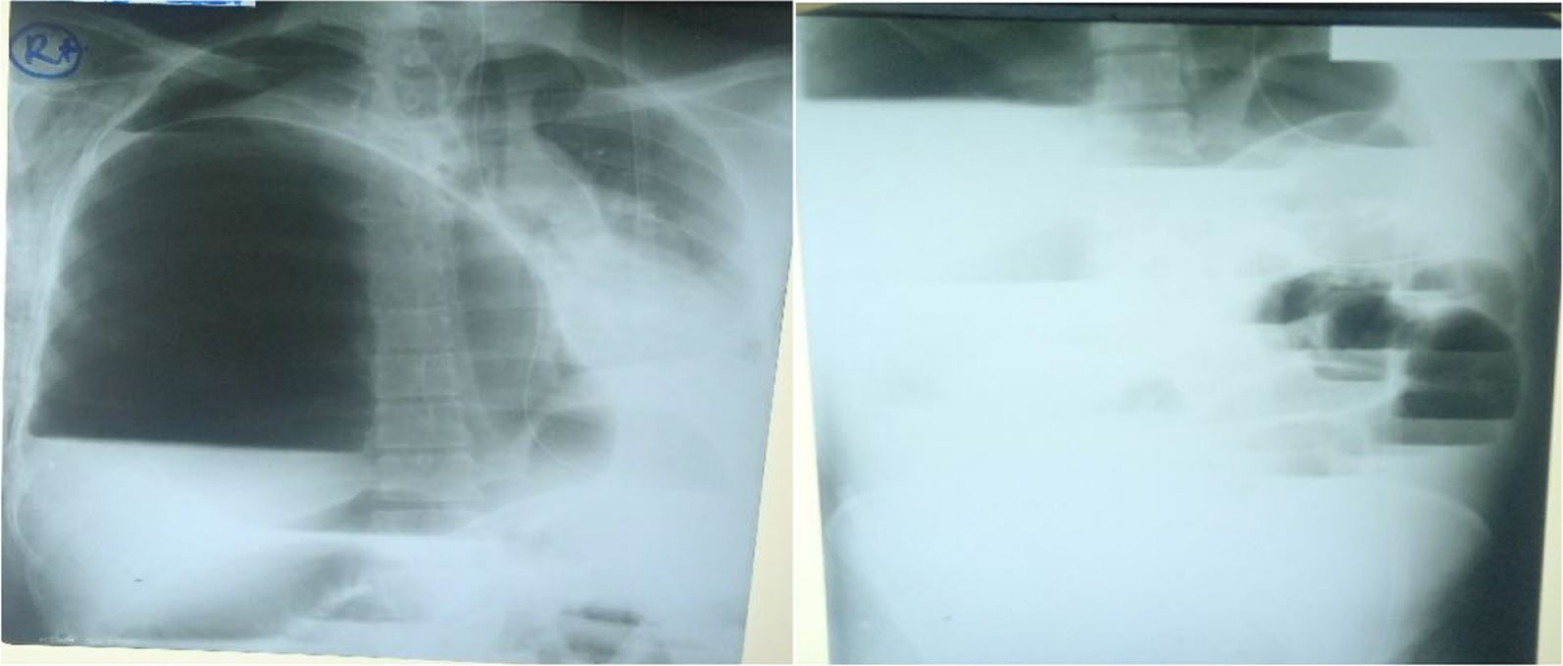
X-rays of the chest and abdomen, respectively, on admission
